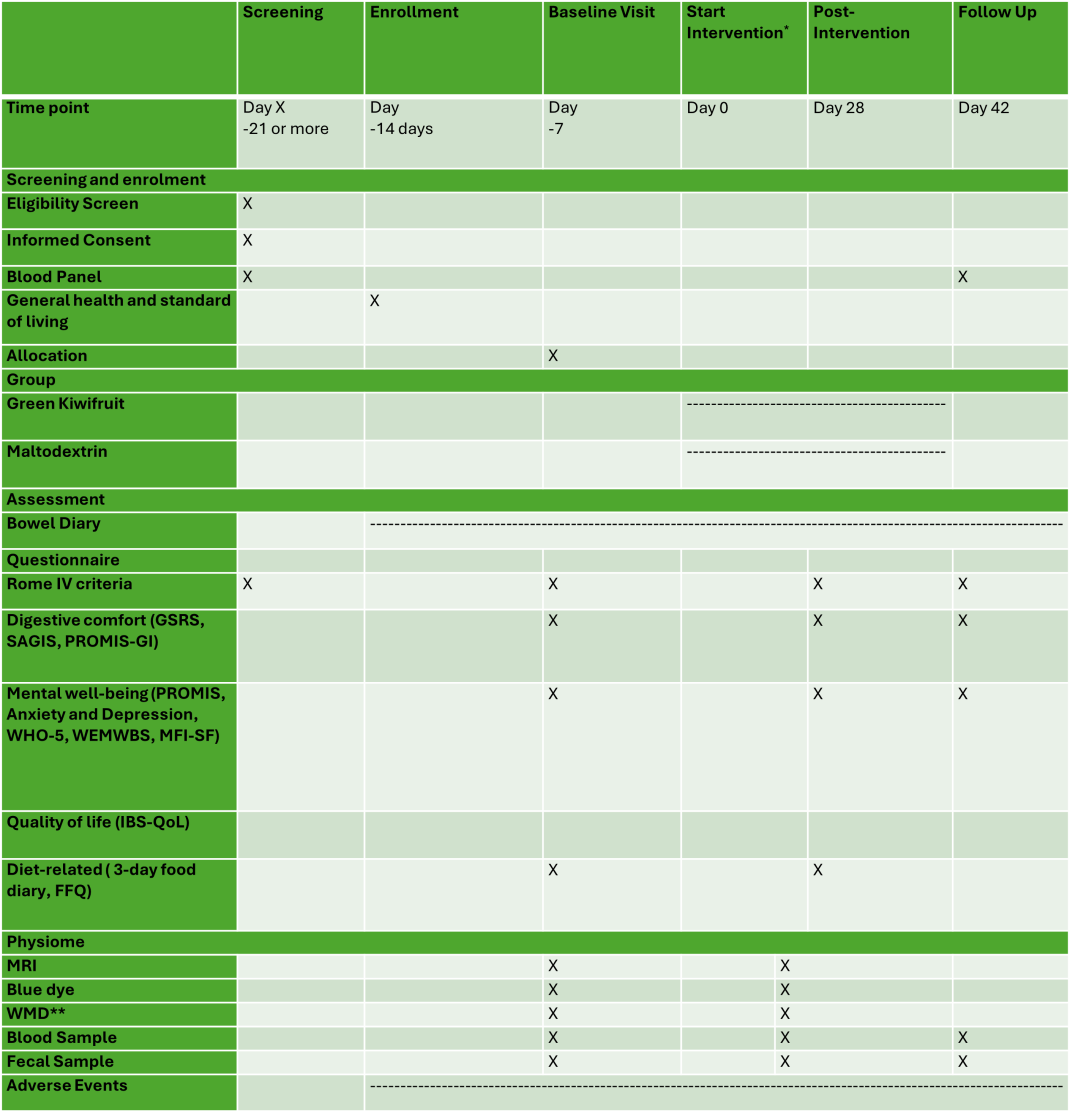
Overview of the schedule of screening, enrollment, interventions, and assessments. FFQ: Food Frequency Questionnaire; GSRS: Gastrointestinal Symptom Rating Scale; IBS-QoL: Irritable Bowel Syndrome Quality of Life Survey; MFI-SF: Multidimensional Fatigue Inventory Short Form; MRI: magnetic resonance imaging; PROMIS: Patient-Reported Outcomes Measurement Information System; PROMIS-GI: Gastrointestinal Domain of the Patient-Reported Outcomes Measurement Information System; SAGIS: Structured Assessment of Gastrointestinal Symptoms; WEMWBS: Warwick-Edinburgh Mental Well-Being Scales; WHO-5: World Health Organization - Five Question Well-Being Index; WMD: wireless motility device. *Participants began intervention 7 days after the baseline visit to ensure the excretion of the WMDwireless motility device.A subset of participants completed this assessment.
